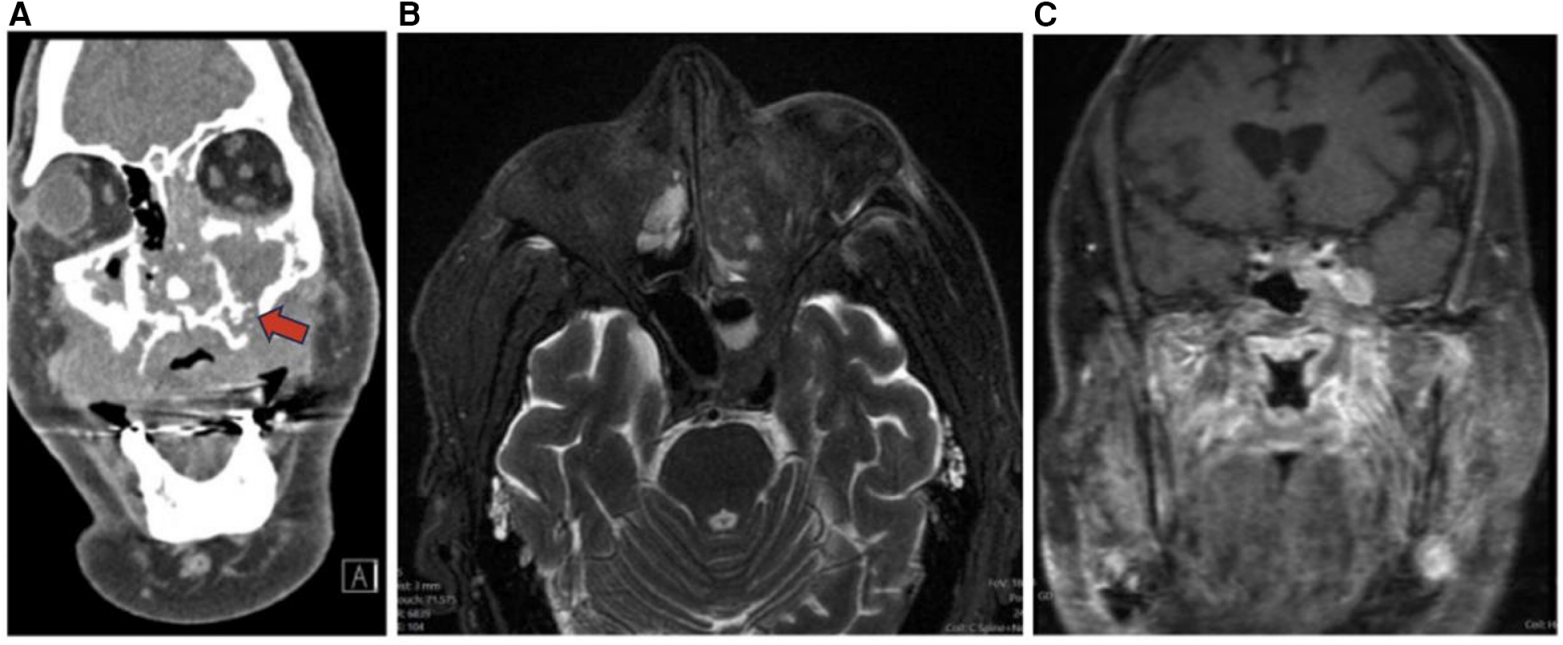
Adenoid cystic carcinoma. Coronal CT (A), axial T2 MRI (B), and coronal T1 + C MRI (C) images demonstrating an adenoid cystic carcinoma centered in the left maxillary sinus. The hard palate demonstrates post contrast enhancement and osseous erosion (red arrow).
